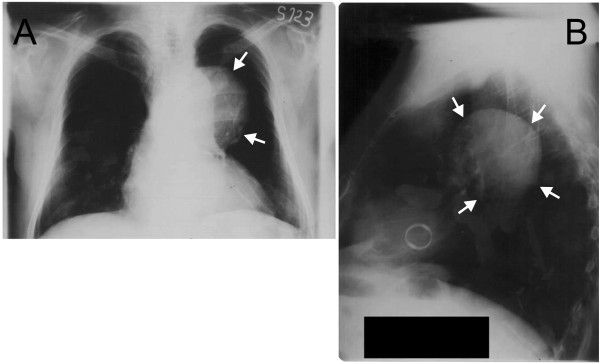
Chest radiography: antero-posterior (A) and left latero-lateral (B).
